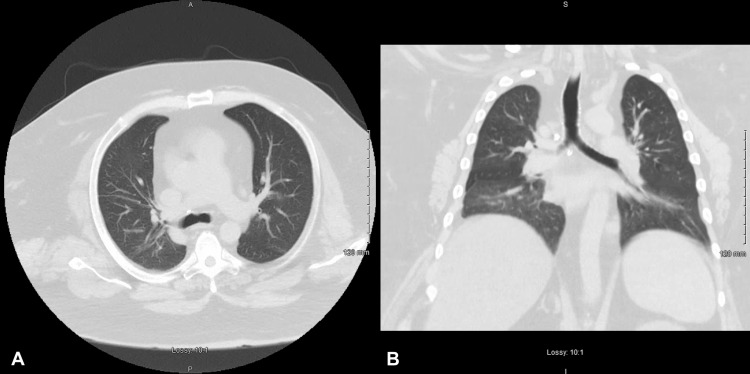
A) axial section of a chest computed tomography demonstrating interval resolution of bilateral pulmonary opacifications; B) coronal section of a chest computed tomography demonstrating interval resolution of bilateral pulmonary opacifications
